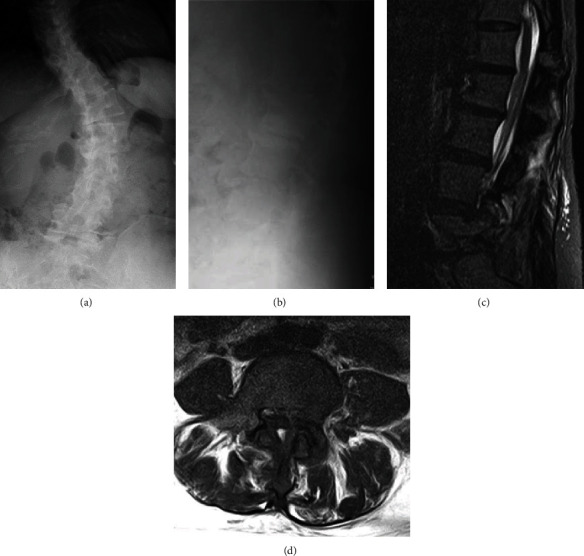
Images of administration on the 5th day of hospitalization. X-ray (a, b) sagittal T2 short tau inversion recovery (T2-STIR) MRI (c), axial L4/5 T2-weighted MRI (d).
